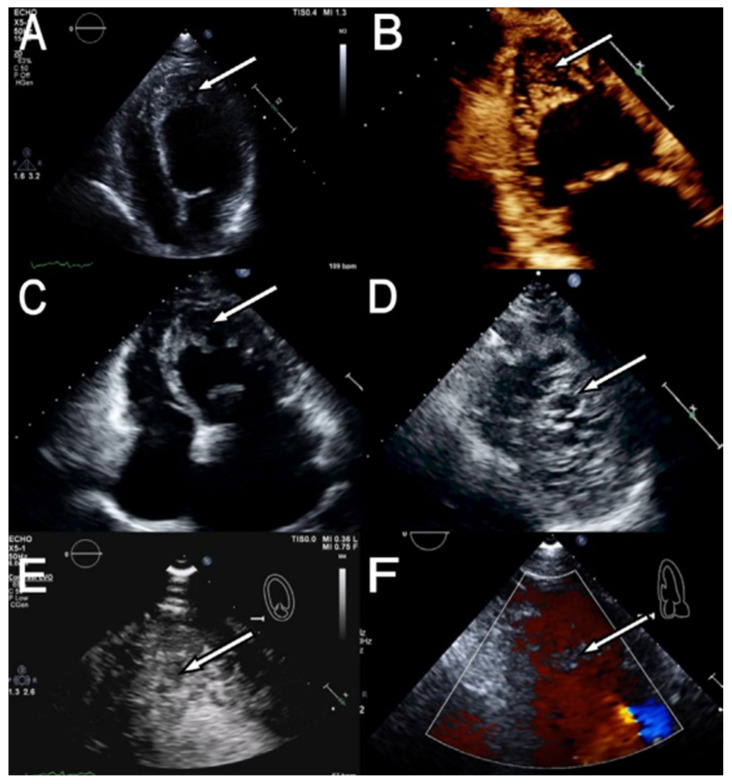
Transthoracic echocardiographic imaging views of a patient with LVNC. (A)—Apical 4-chamber view showing apical noncompaction (*arrow*). (B)—Apical 2-chamber view showing apical noncompaction (*arrow*). (C)—Apical 4-chamber view showing LVNC (*arrow*). (D)—Parasternal short-axis view at the level of the LV apex showing noncompaction (*arrow*). (E)—High magnification of LV apex showing noncompaction highlighted by contrast (*arrow*). (F)—Color Doppler flow within intertrabecular recesses shown in LVNC (*arrow*). *Source: Rao, K., Bhaskaran, A., Choudhary, P., & Tan, T. C. (2020). The role of multimodality imaging in the diagnosis of left ventricular noncompaction. European Journal of Clinical Investigation, 50(9), e13254* [55].
